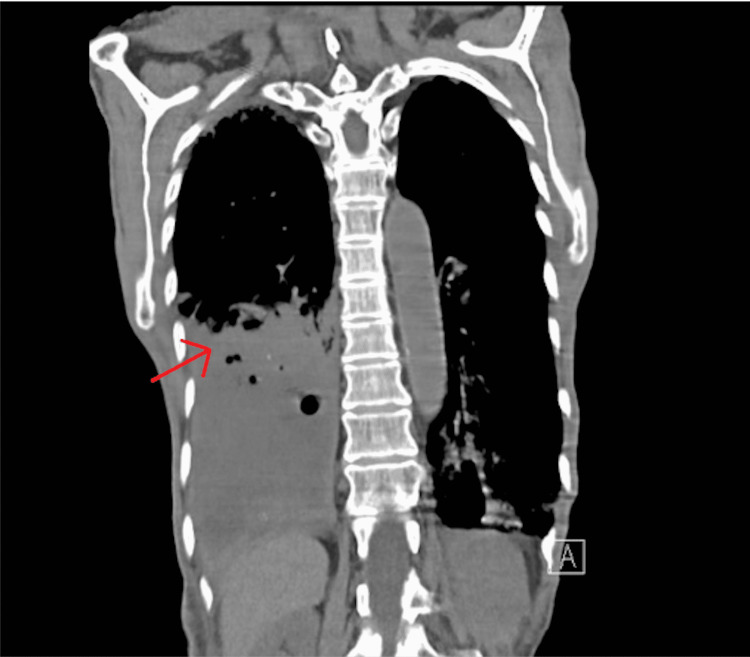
Coronal CT scan without contrast identifying bilateral pulmonary infiltrates with a large air-fluid collection (pointed to by the red arrow) within the right lobe
